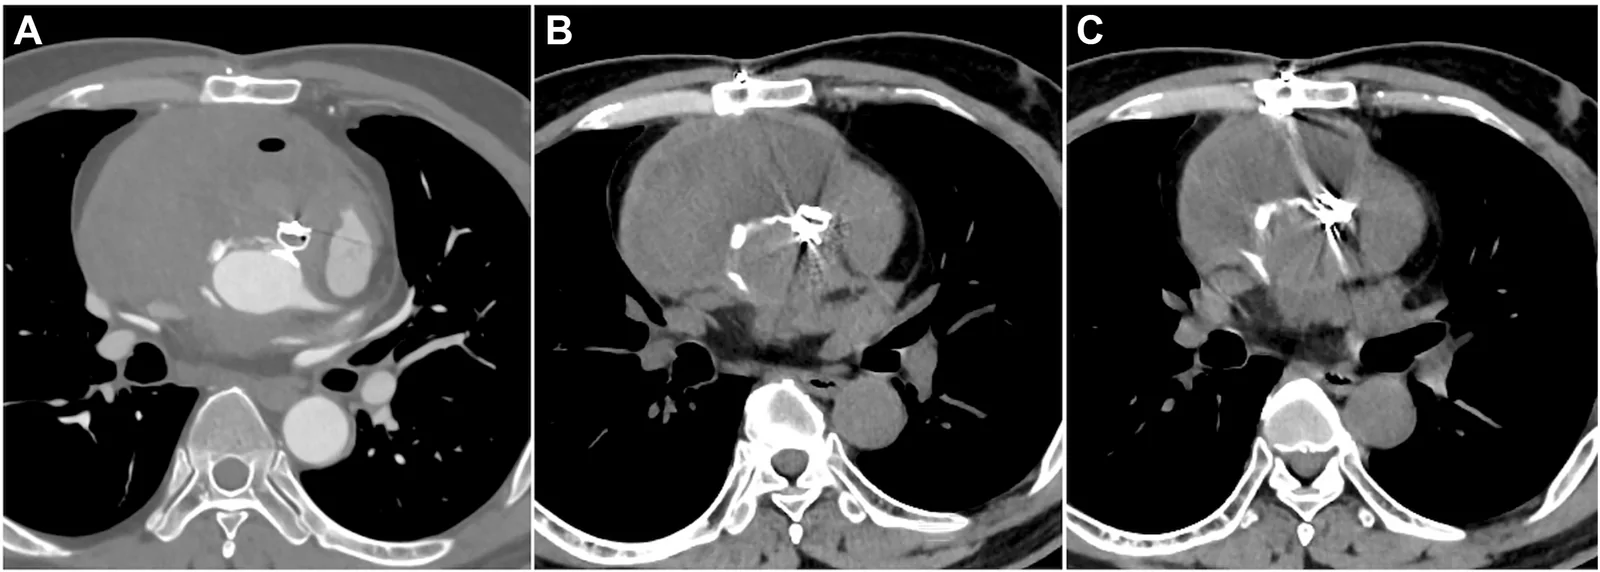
Serial computed tomography images after plug closure. (A) Immediately after the procedure, no residual leak or device migration is observed; the pseudoaneurysm measured 108 × 56 mm. (B) At 3 months, an interval reduction of the pseudoaneurysm (101 × 48 mm) is noted. (C) At 6 months, further shrinkage to 85 × 40 mm is evident, demonstrating progressive involution over time.
